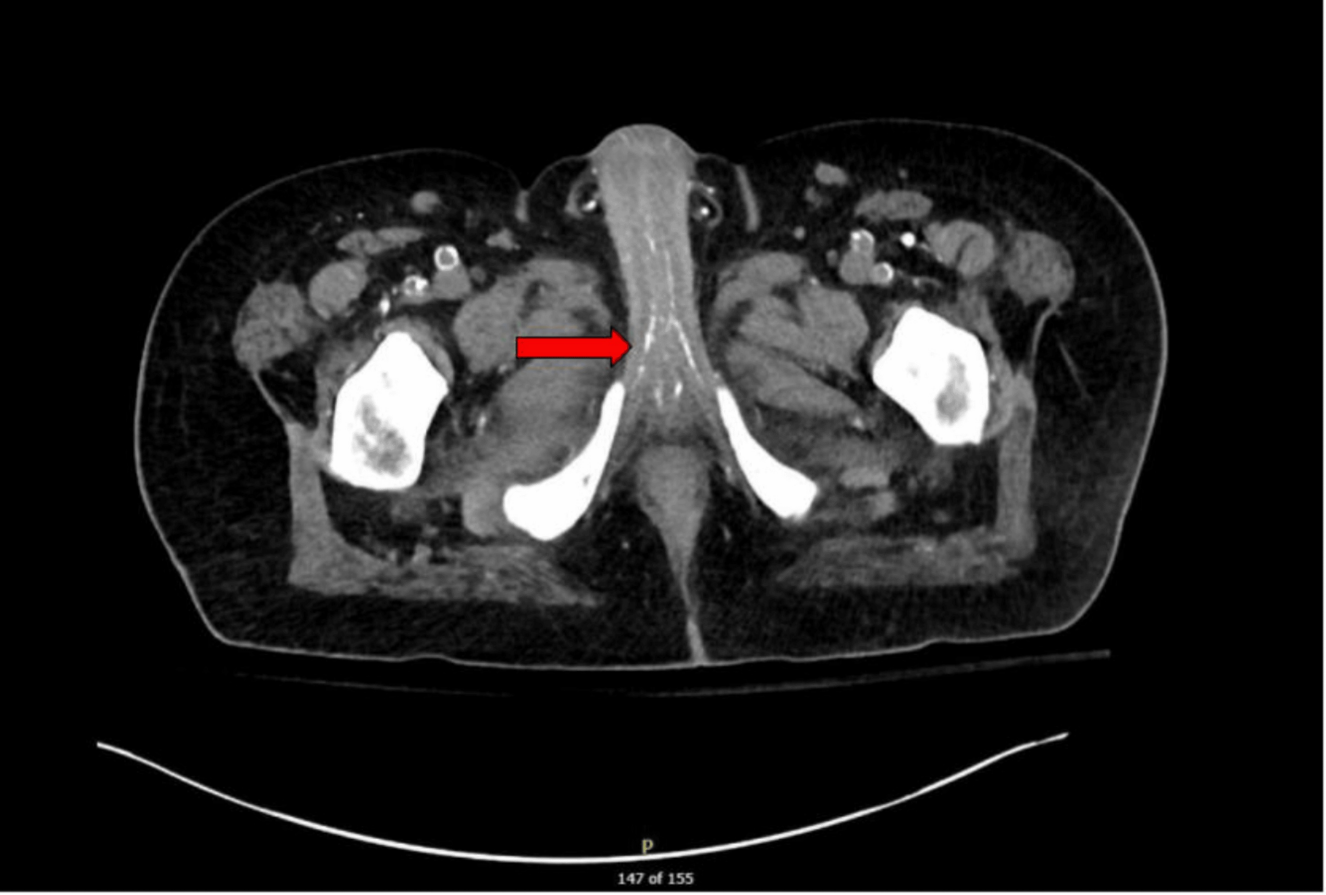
CT abdomen pelvis without IV contrast Axial image delineating extensive calcification of the cavernosal arteries (red arrow)
